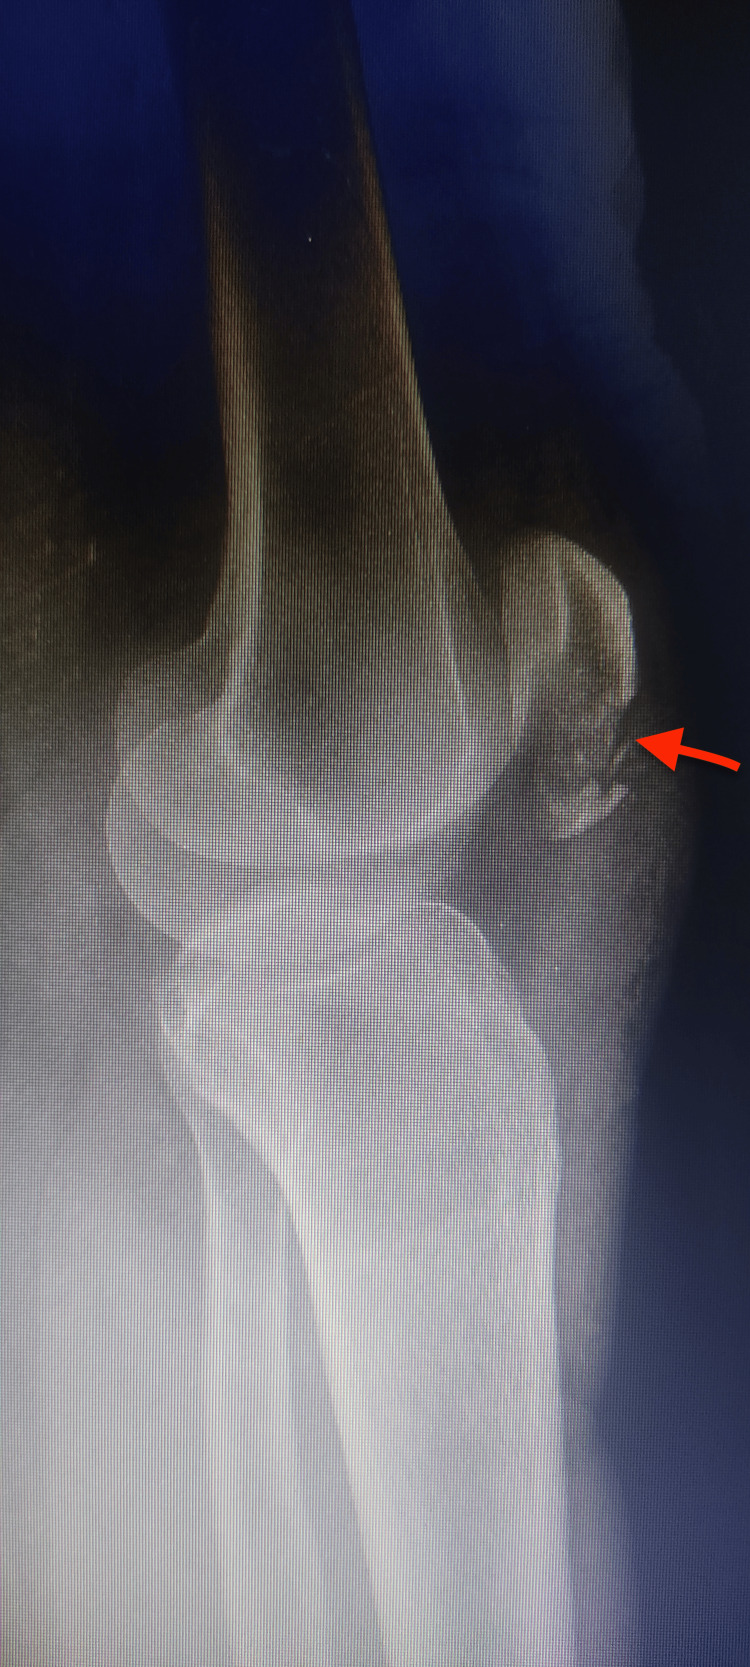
Lateral radiograph of the left knee showing a comminuted inferior pole patellar fracture.
